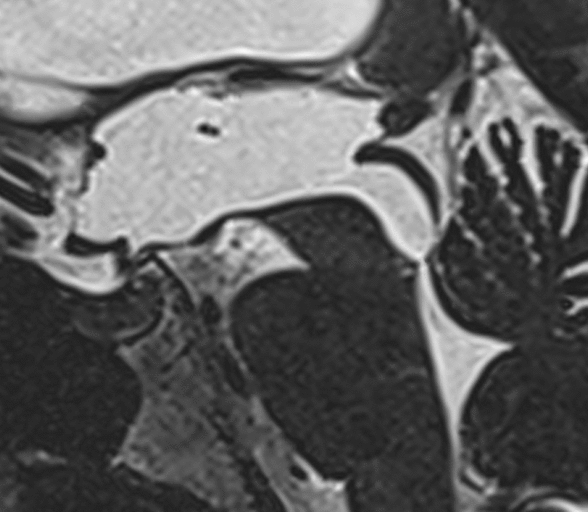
Sagittal T2 MRI image through the third ventricle, cerebral aqueduct and fourth ventricle showing typical findings for LIAS. Note the convex shape of the tuber cinereum and lamina terminalis, dilated third ventricle, stenosis of the distal aqueduct and normal sized fourth ventricle
